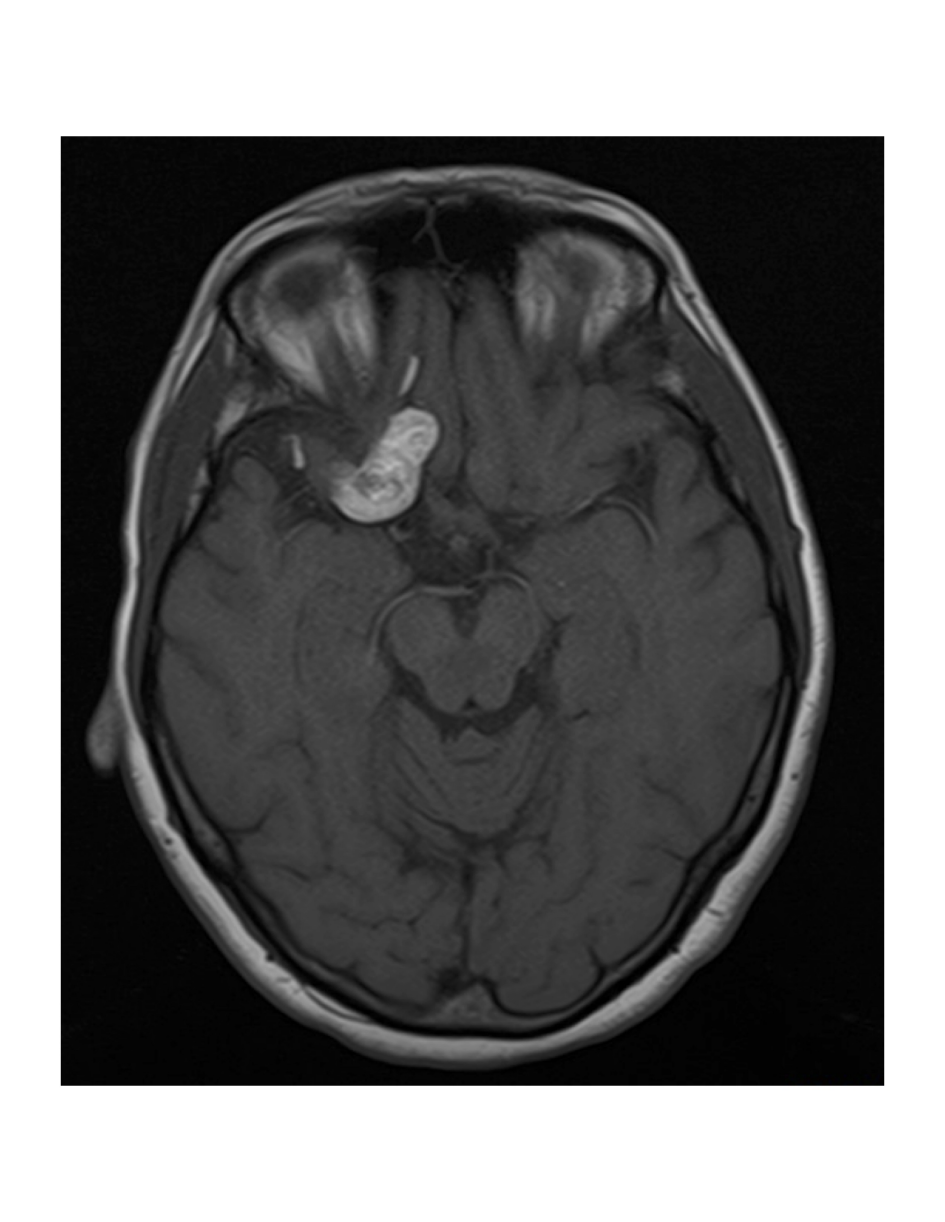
2009 Repeat MRI Repeat T1-weighted MRI image in 2009 showing no new evidence of significant change in appearance.
